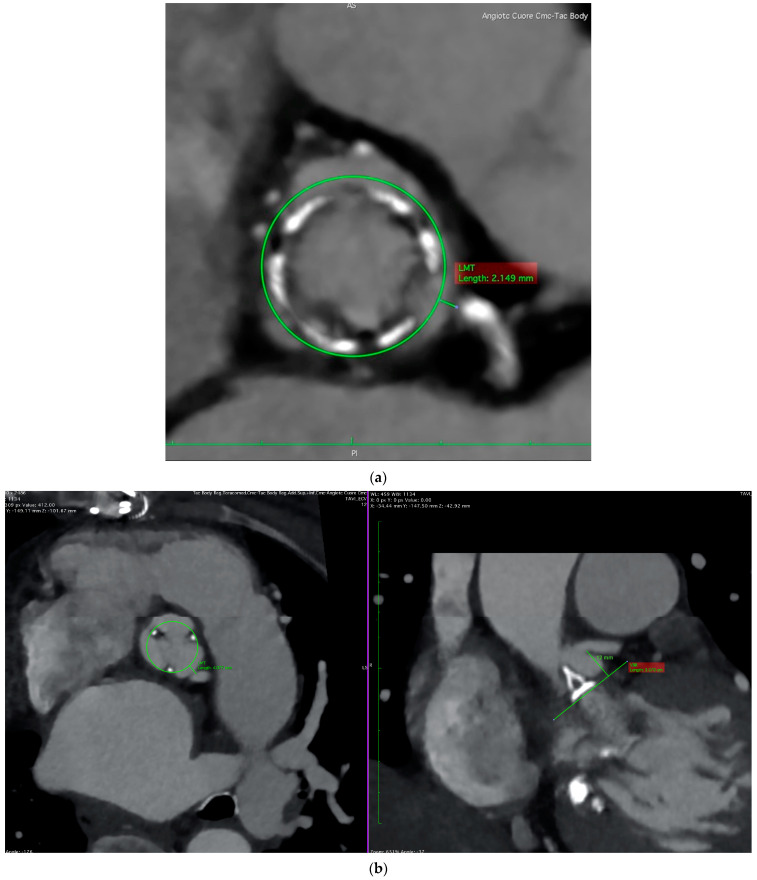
Cardiac computed tomography. Panel (a): valve to coronary (left main) distance < 4 mm, a typical case of a patient at high risk for coronary obstruction. Panel (b): although valve to coronary distance (4.08 mm) depicted this patient at intermediate risk for obstruction, the concomitant height of the coronary (12 mm) was adequate for safely performing ViV procedure.
